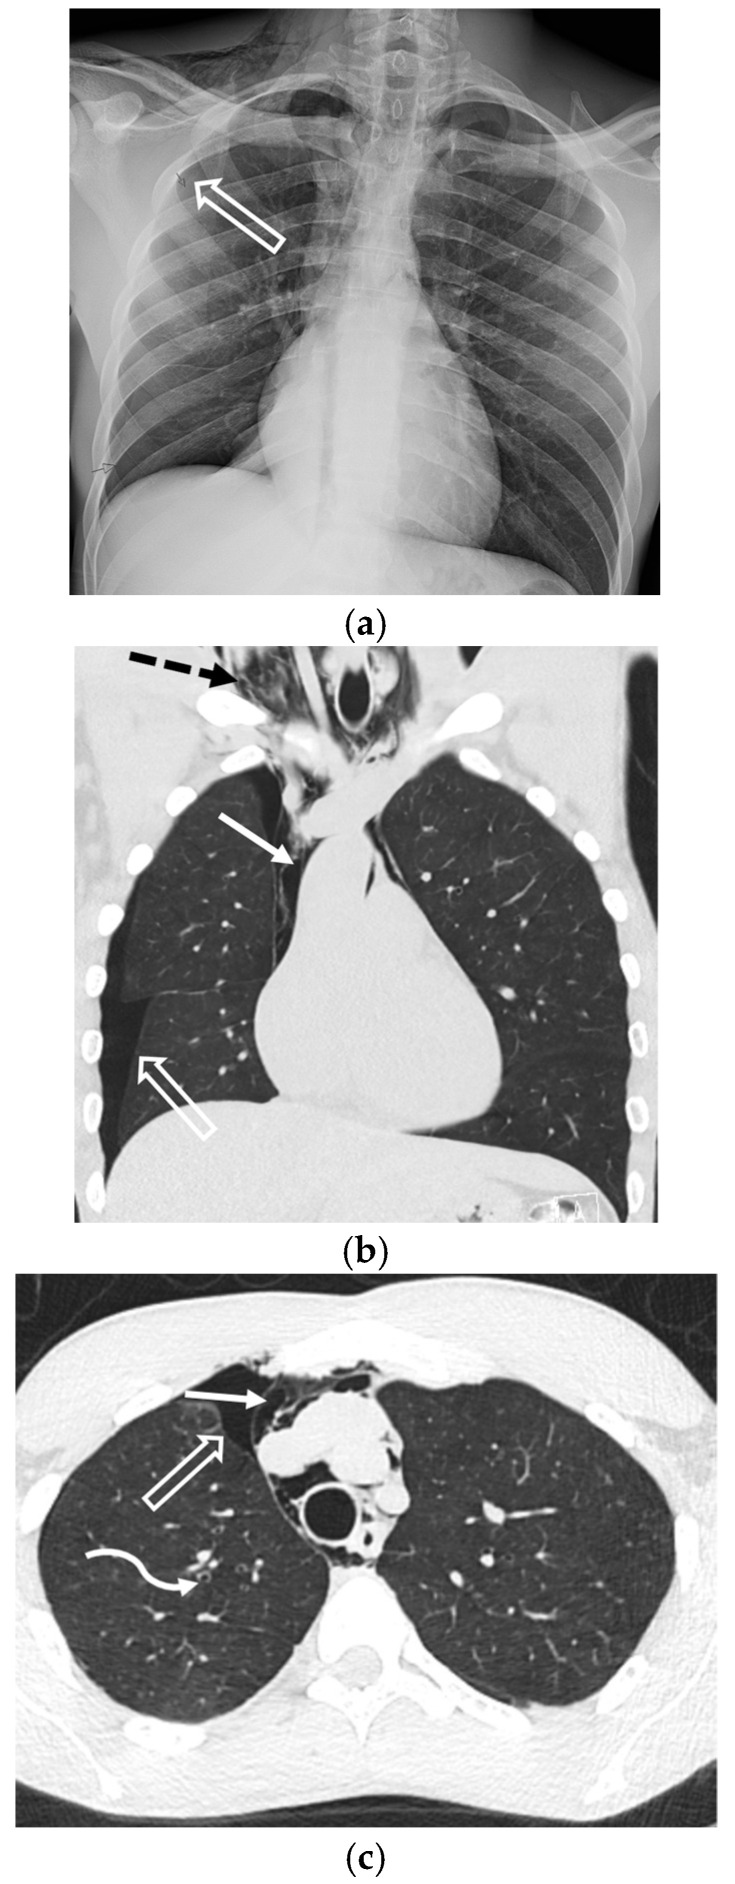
28-year-old male with asthma presents with chest pain. Frontal chest radiograph (a) demonstrates small-to-moderate right pneumothorax (open white arrow). There is also pneumomediastinum (solid white arrow) and subcutaneous emphysema (dashed black arrow), better evaluated on coronal and axial CT images in lung window (b,c). Axial CT image also shows diffuse bronchial wall thickening (curved white arrow) due to bronchitis.
